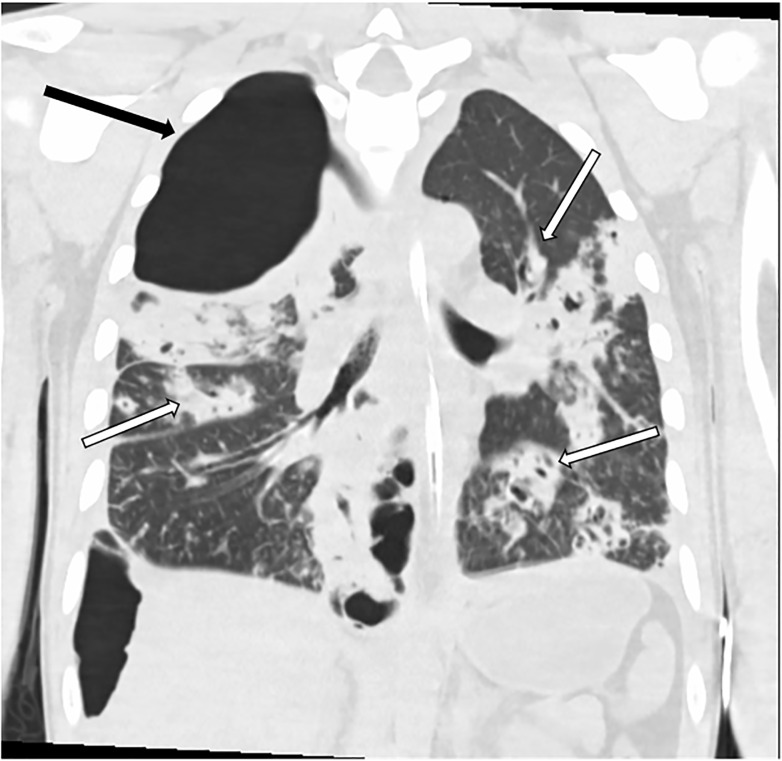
Thorax computed tomography coronal plane demonstrating right upper lobe gigantic bulla (black arrow) as well as multifocal pneumonia with bronchiectasis (multiple white arrows).
